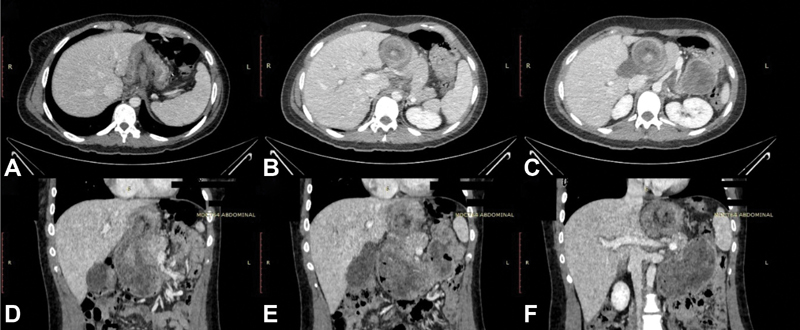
CT scanner of the abdomen and pelvis with intravenous contrast showing abnormal displacement of the entire stomach to posterior of the SMA and SMV. The antrum is shown on the left side consistent with mesenteroaxial gastric volvulus. The GEJ is shown inferior to the antrum and the pancreas has an inverted
